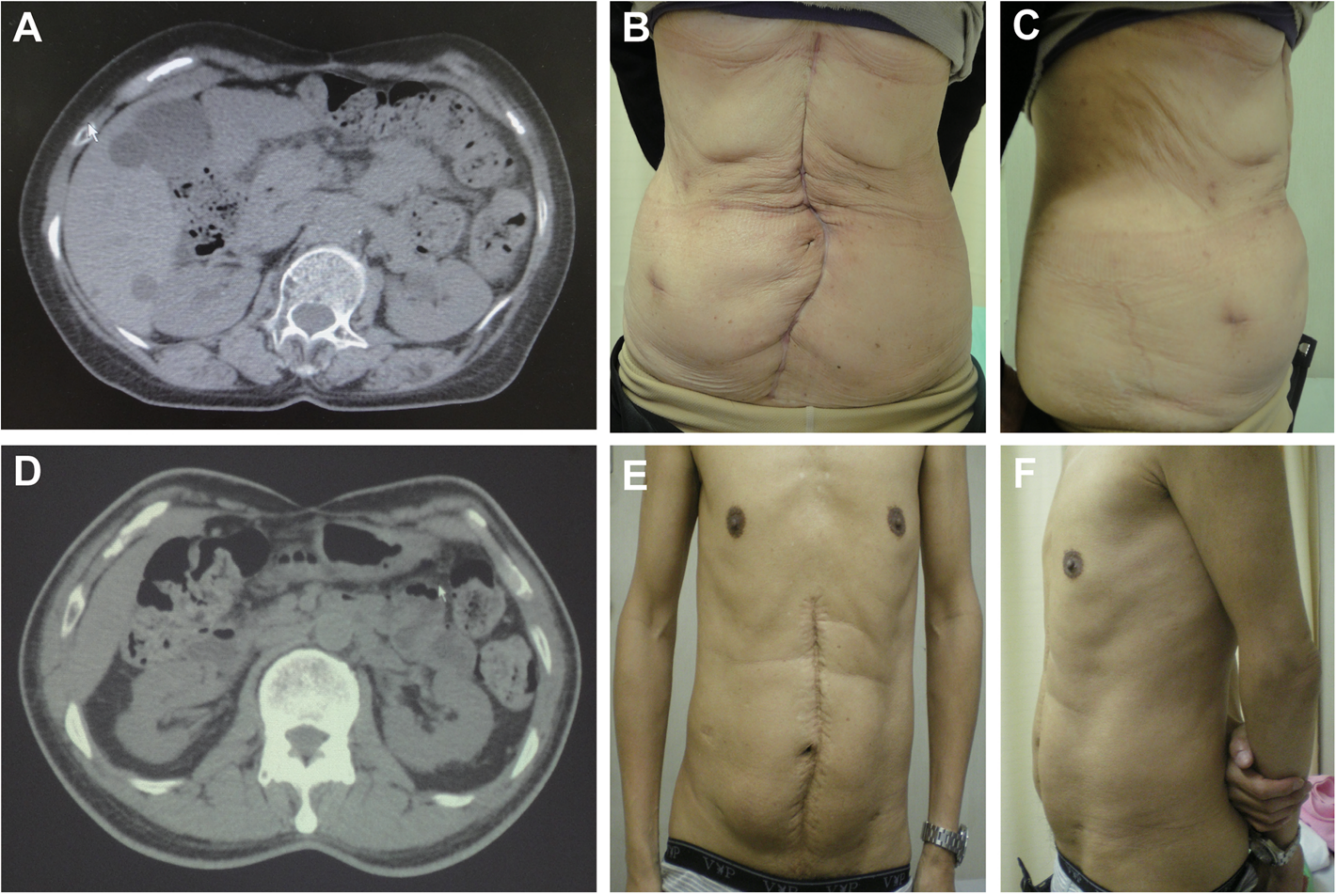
The CT findings and views of the abdominal wall. The upper panels show images obtained 6 years after performing the turnover flap method (a, b, c). The lower panels show images obtained 10 years after turnover flap method (d, e, f). The CT scans show that the appearance of flaps created using bilateral anterior rectus abdominis sheath looks similar to after onlay mesh repair (a, d). Anteroposterior views (b, e) and lateral views (c, f) of the abdominal wall in the standing position. Although slight lower abdominal bulging can be observed in the lateral views of both patients, no abdominal hernia is evident
